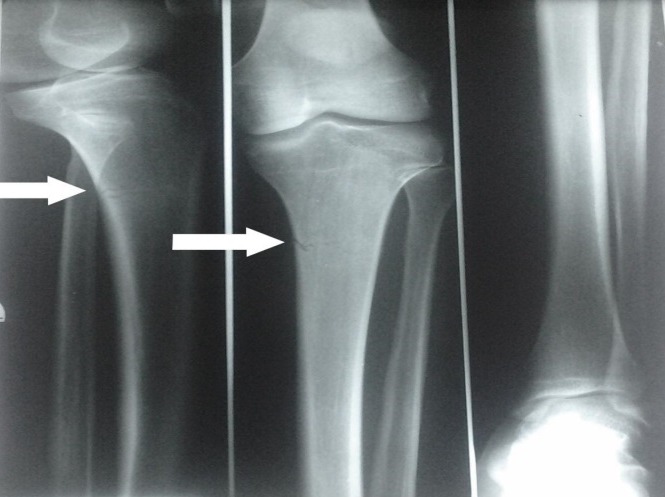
Jeune recrue de 20 ans présentant une douleur du tiers supérieur de la jambe gauche après une marche de 25 Km: radiographie de la jambe gauche de face et de profil montrant une fracture mixte corticale et spongieuse du tiers supérieur de la diaphyse tibiale
